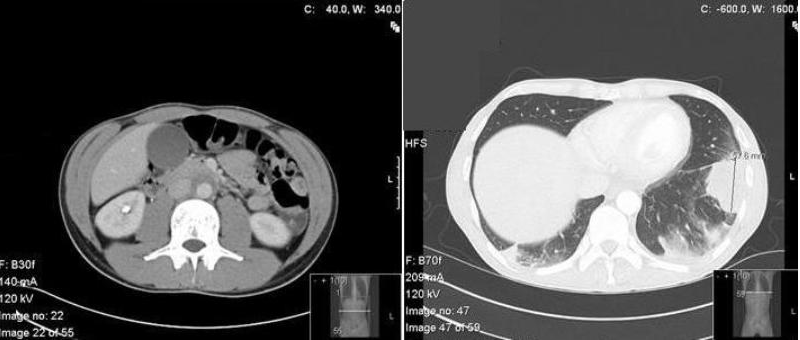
(A) CT scan of abdomen showing a localised abdominal aortic aneurysm. (B) CT scan of the chest showing lung nodules at the left lower lobe.
